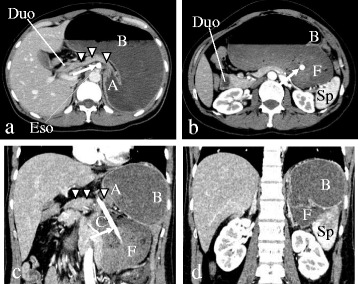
Contrast-enhanced abdominal CT findings. The *arrow* shows the NG tube inserted into the stomach. Axial CT scan at the abdominal esophagus level (a) demonstrates the grossly enlarged stomach with resultant displacement of the gastric antrum (*A* and *arrowheads*) above the abdominal esophagus. More caudal axial CT scan (b) and coronal CT images (c, d) reveal the stomach to be twisted mesenteroaxially, with the antrum (*A*) positioned higher than the fundus (*F*). CT findings (b, d) show the normal-sized spleen positioned inferiorly toward the left kidney as compared to its normal position. *CT* computed tomography, *NG tube* nasogastric tube, *A* antrum, *B* body, *C* cardia, *Duo* duodenum, *Eso* esophagus, *F* fundus, *Sp* spleen
